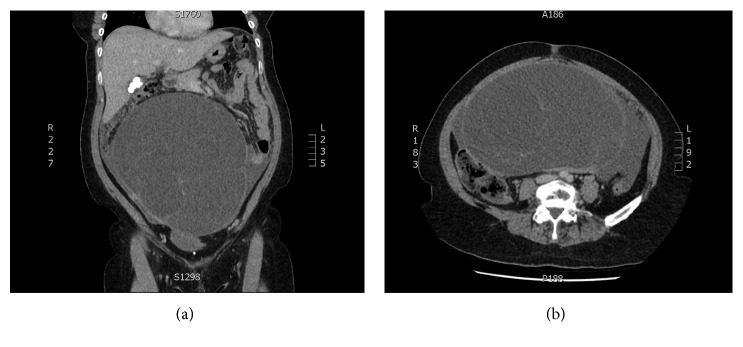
(a, b) Follow-up CT showing ruptured ovarian cyst.
